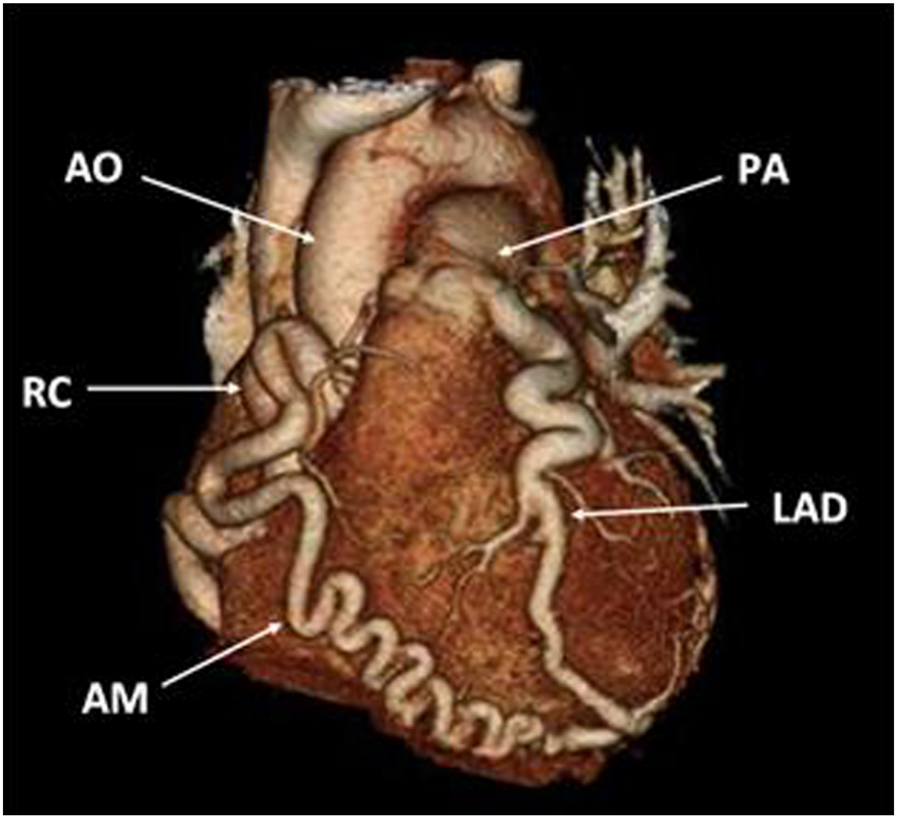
CTA 3D reconstruction of the heart. Aorta (AO), Right coronary artery (RC), acute marginal (AM), pulmonary artery (PA) and left anterior descending artery (LAD).
